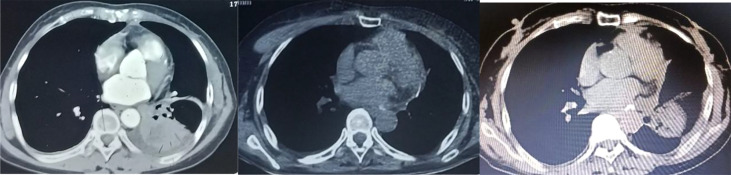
Chest CT contrast (From left to right:2019.1-2021.6-2022.4).
